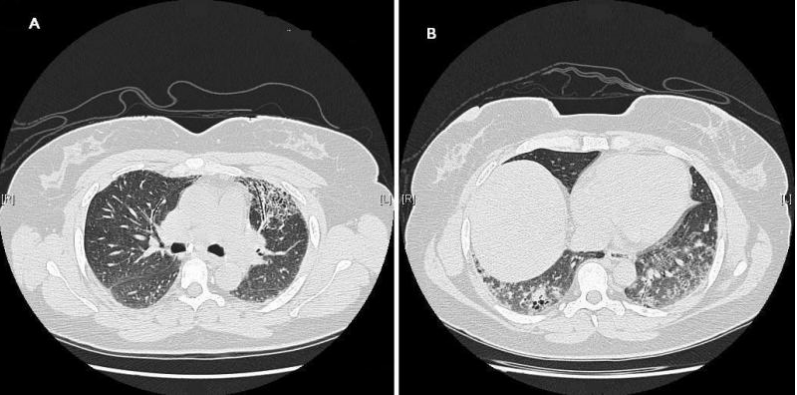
Case 2. (a) HRCT scan obtained at the upper lung zones shows reticular opacities and honeycombing. (b) HRCT scan from the basal parts of the lungs showing ground-glass attenuation, reticular and nodular densities, and traction bronchiectasis.
